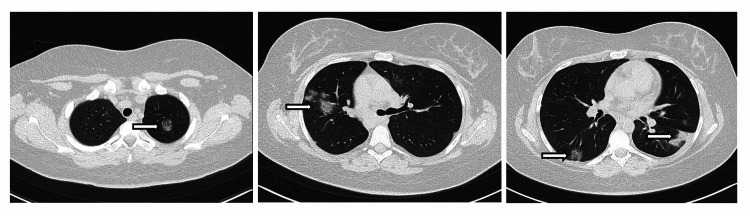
CT chest a month after discharge showing significant improvement in previously noted pulmonary consolidations. New bilateral patchy opacities were noted. Left: upper lung zones, middle: mid lung zones at the level of the left main bronchus, right: lower lung zones. White arrows are pointing to the new pulmonary opacities.
